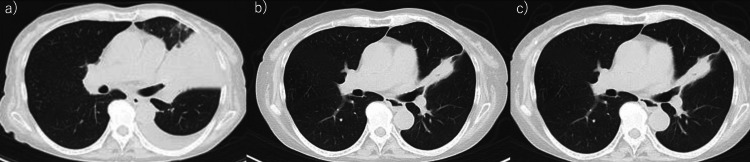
Computed tomography scans show the patient’s course during left upper lobe small-cell lung cancer. In her left upper lobe, the small-cell lung cancer mass continued to shrink after chemotherapy was discontinued. (a) First visit to our hospital. (b) First visit to the Neurology department. (c) Second visit to the Neurology department after treatment with steroids and intravenous immunoglobulin therapy.
